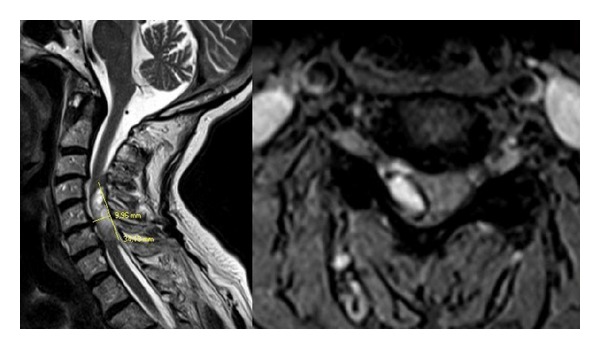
MRI of spine showed epidural hematoma lining right lateral to spinal cord in cervical region.
